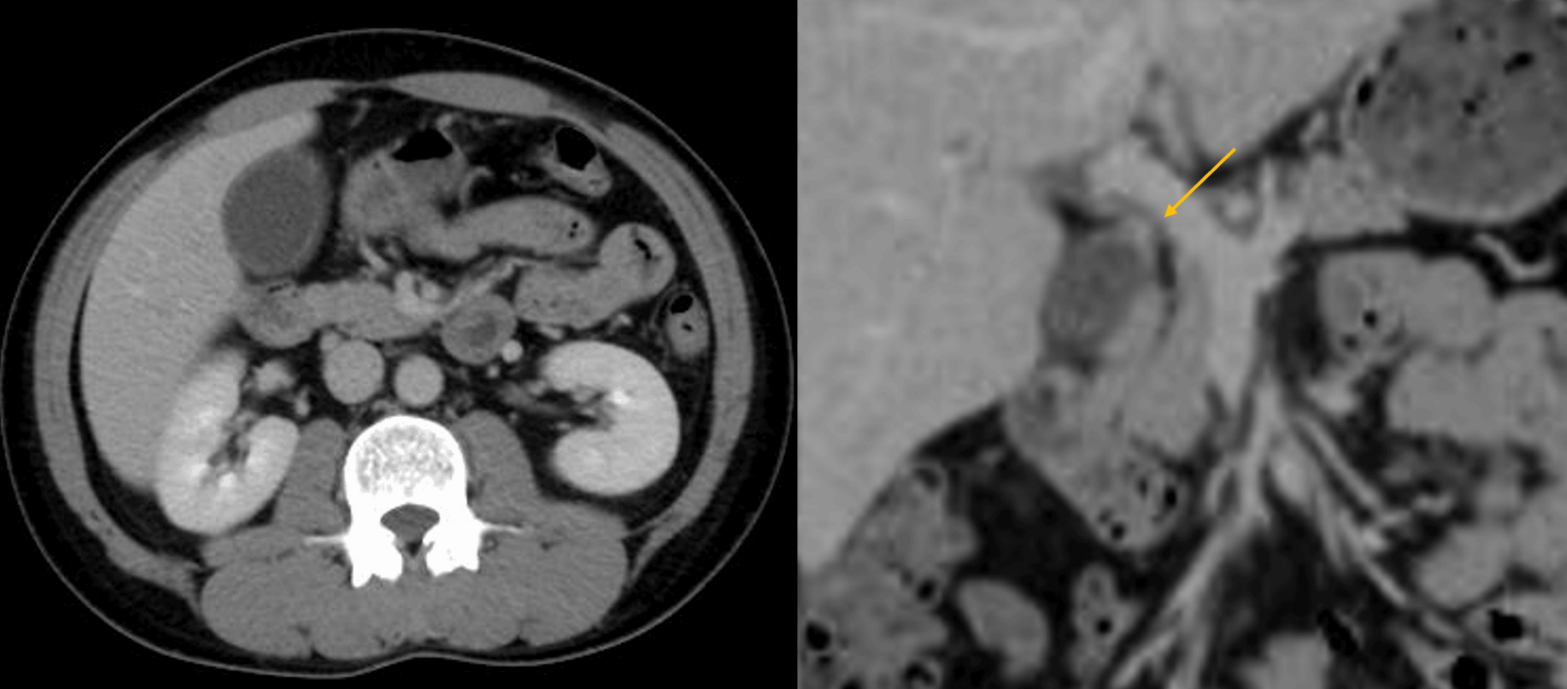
Findings of Abdominal CT Abdominal CT demonstrated gallbladder distension and wall thickening and revealed a mildly enhancing mass at the cystic duct junction (arrow).
